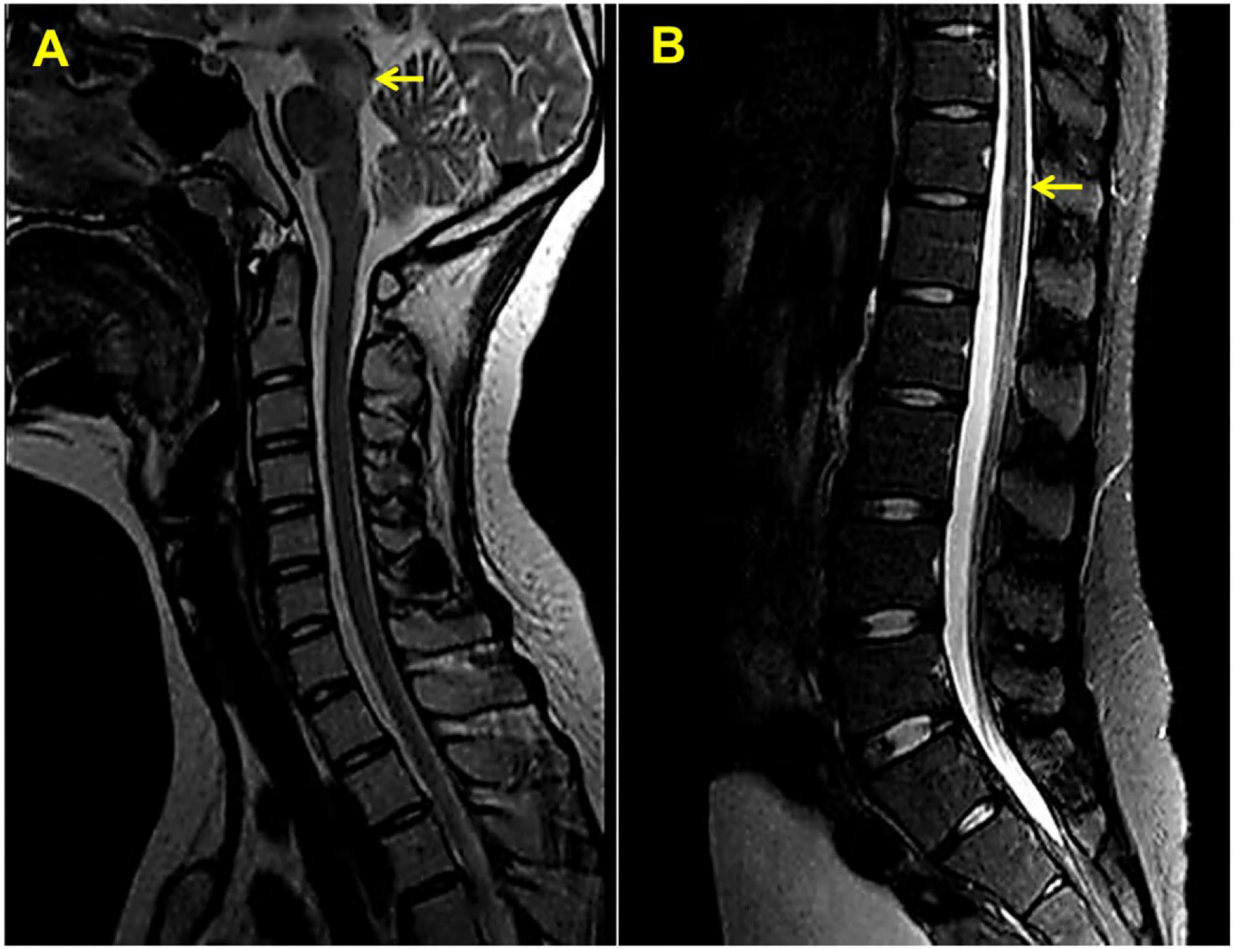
Sagittal T2-weighted magnetic resonance imaging of the upper (A) and lower (B) spinal cord. Yellow arrows indicate white matter lesions.
